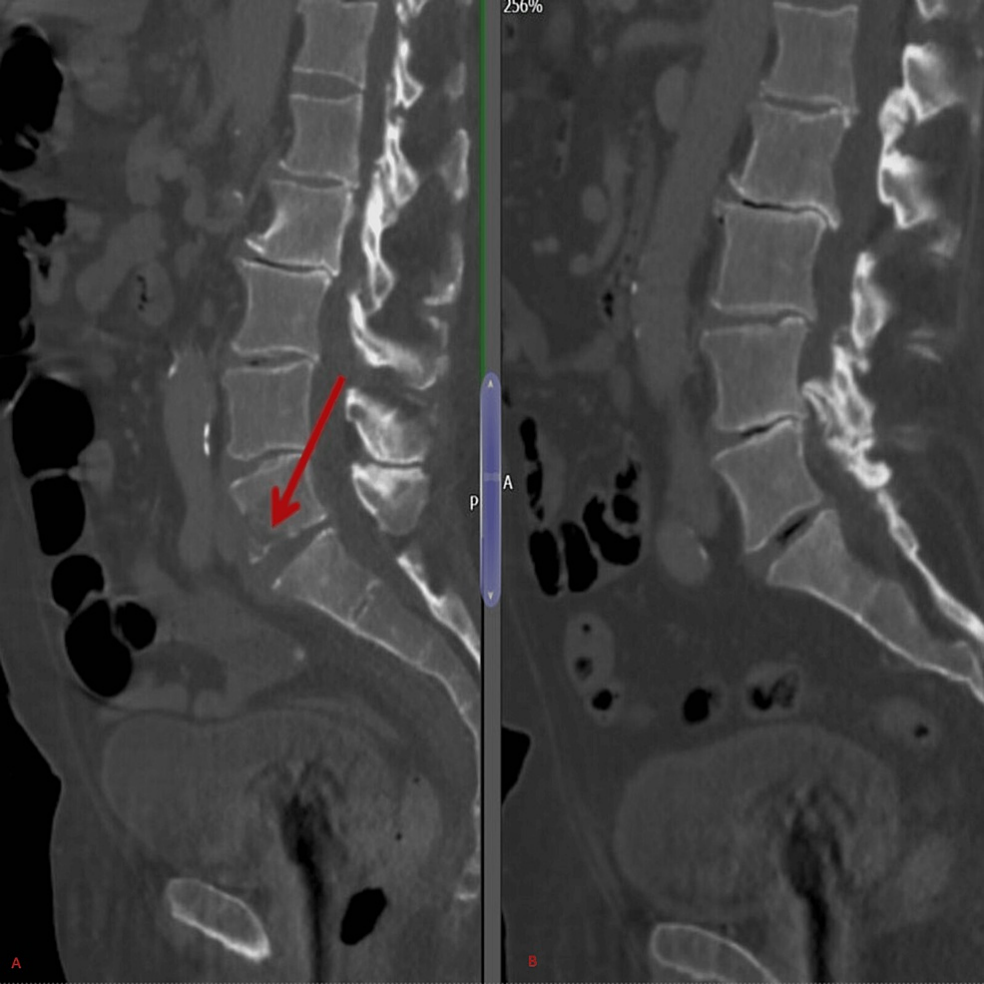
CT abdomen sagittal view showing an Ill-defined bone lesion (arrow) in L5 vertebral body (A) compared to a previous scan the patient had few months prior to admission (B)
